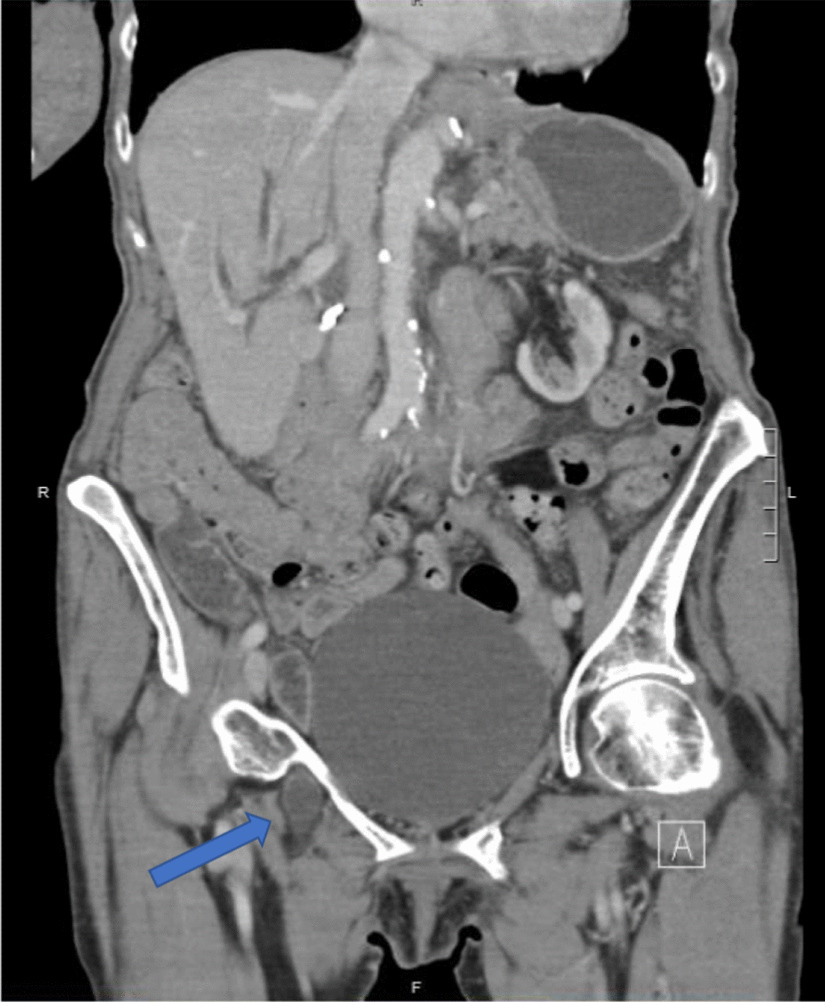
Coronal computed tomography scan showing a right-sided obturator hernia (denoted by the blue arrow)
